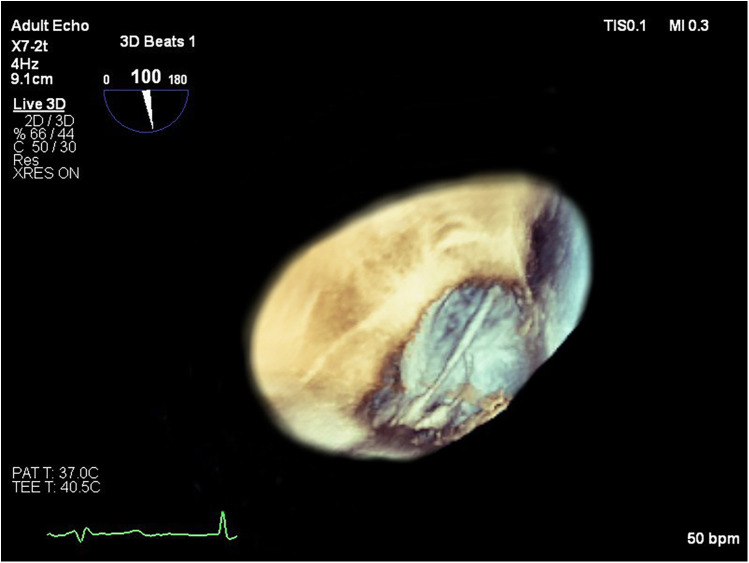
Short-axis left ventricular transesophageal 3D echocardiographic view showing the transit of the two pacing leads across the atrial septal defect. 3D, three-dimensional.
